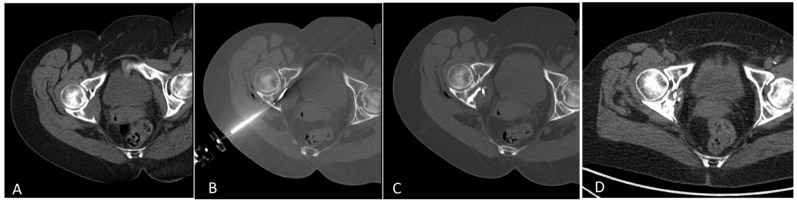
Treatment with tRFA and osteoplasty of an osteolytic metastasis of the posterior column of the right hip from breast cancer in a 65-year-old woman (A). Axial CT showed the placement of the steerable radiofrequency bipolar device (tSTAR) (B). Axial CT scan showed the metastases filled by PMMA (C). Axial CT after 12 months showed a partial recalcification of the posterior column of the hip and disappearance of the tumor mass (D).
